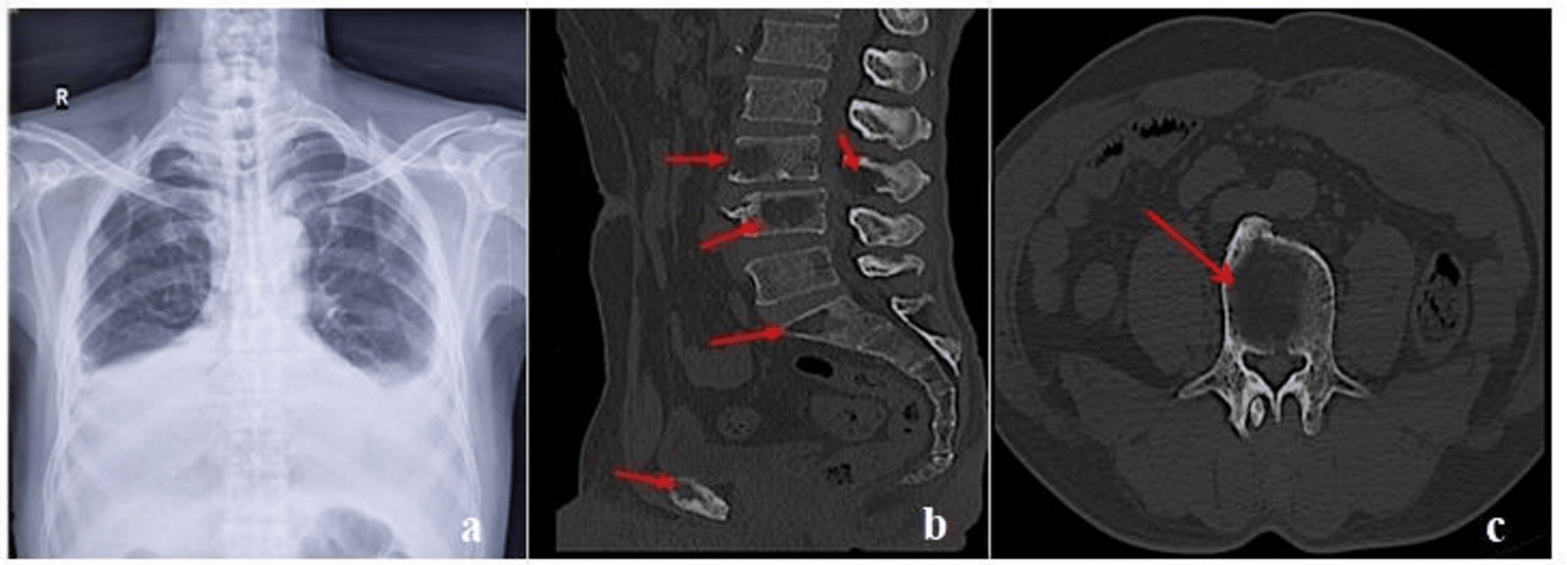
Chest X-ray showing evidence of bilateral pleural effusion. Sagittal CT of lumbar sacral spine in bone window (a). Multiple lytic lesions in the L4, L5, and S1 vertebral bodies, L3 spinous process, and superior pubic ramus (red solid arrow) (b). Axial CT of lumbar sacral spine in bone window showing lytic lesion in the L4 vertebral body (red solid arrow) (c) CT: computed tomography
